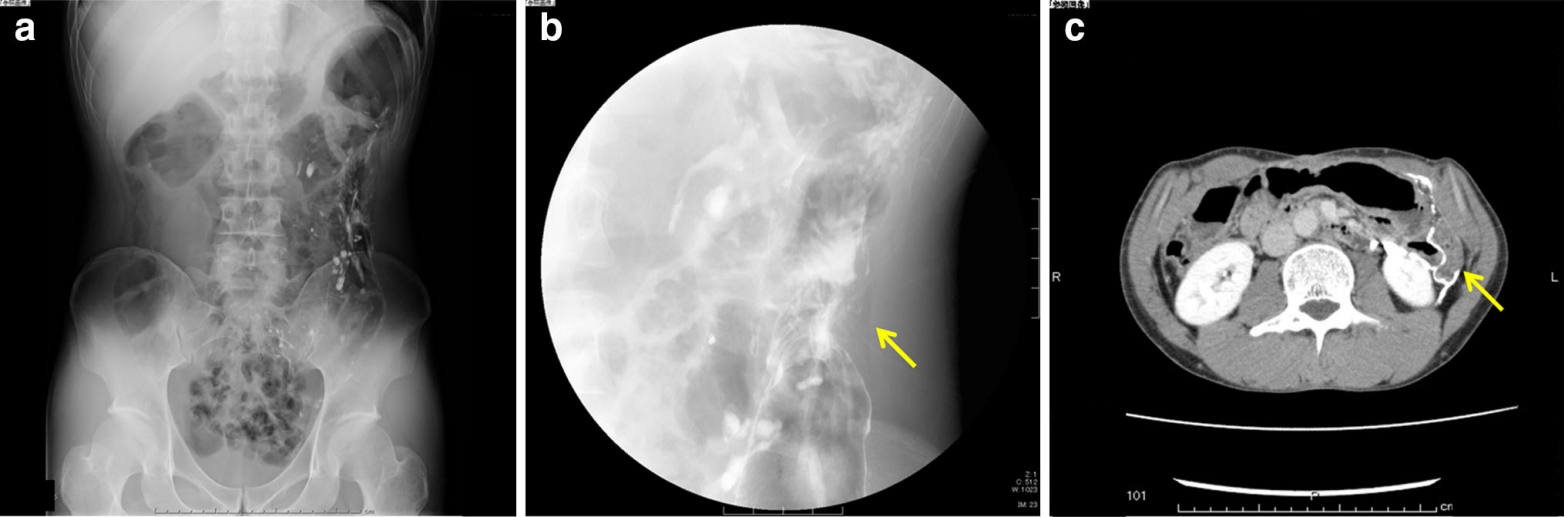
a Radiography, b gastrografin enema examination, and c computed tomography (CT) revealed stenosis of the descending colon (*arrows*) and remarkable distention on the oral side of the descending colon. Barium nodules were detected in the peritoneal cavity, especially near the anastomosis
